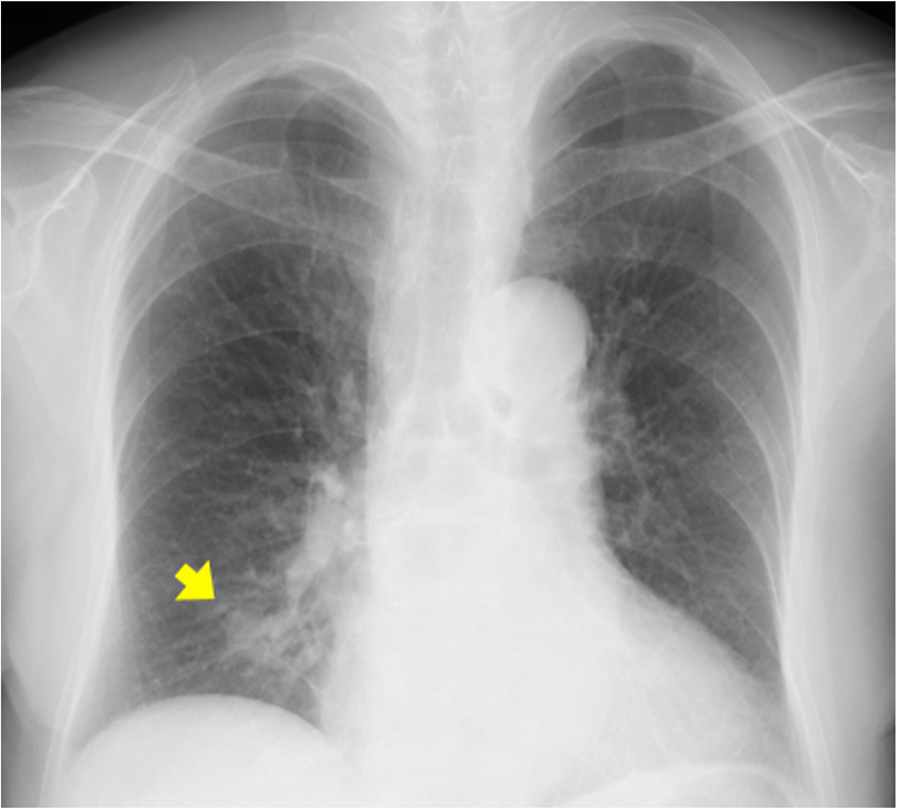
Chest X-ray shows an abnormal shadow in the right lower lung field. The lesion is indicated by the arrow.
